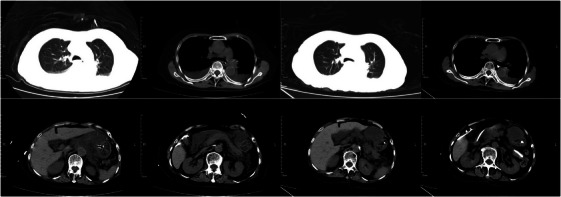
Systemic computed tomography (CT) imaging changes associated with sepsis. Imaging changes before and after treatment in an 81‐year‐old female patient with sepsis (severe acute pancreatitis [SAP] and biliary tract stones).
